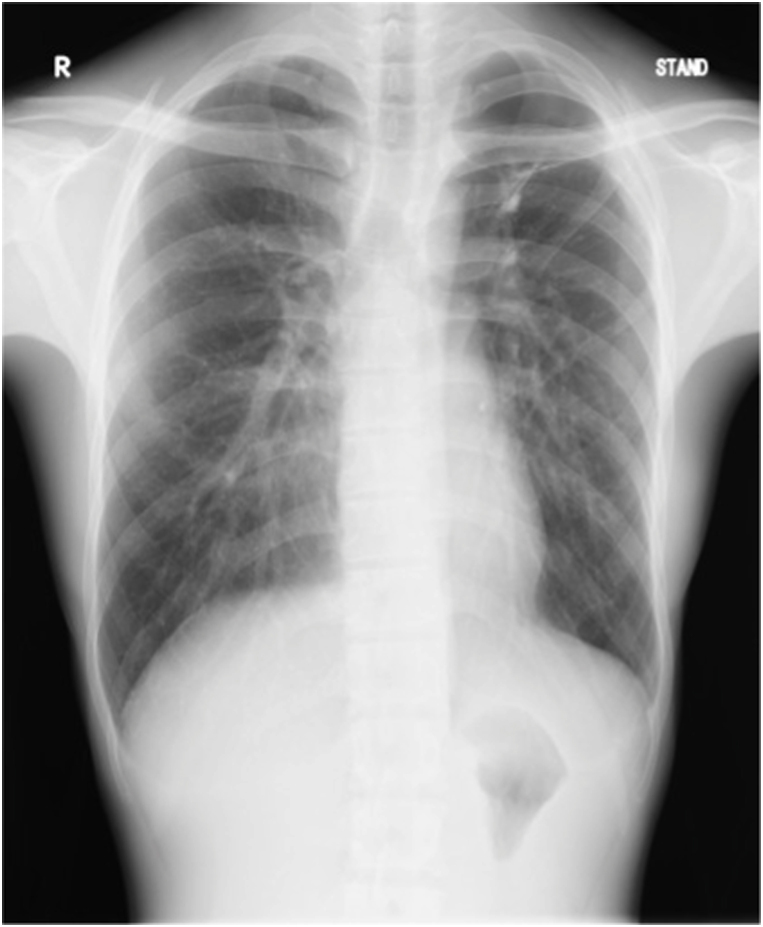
Chest radiography one year after the operation showed full reexpansion of the left lung.
